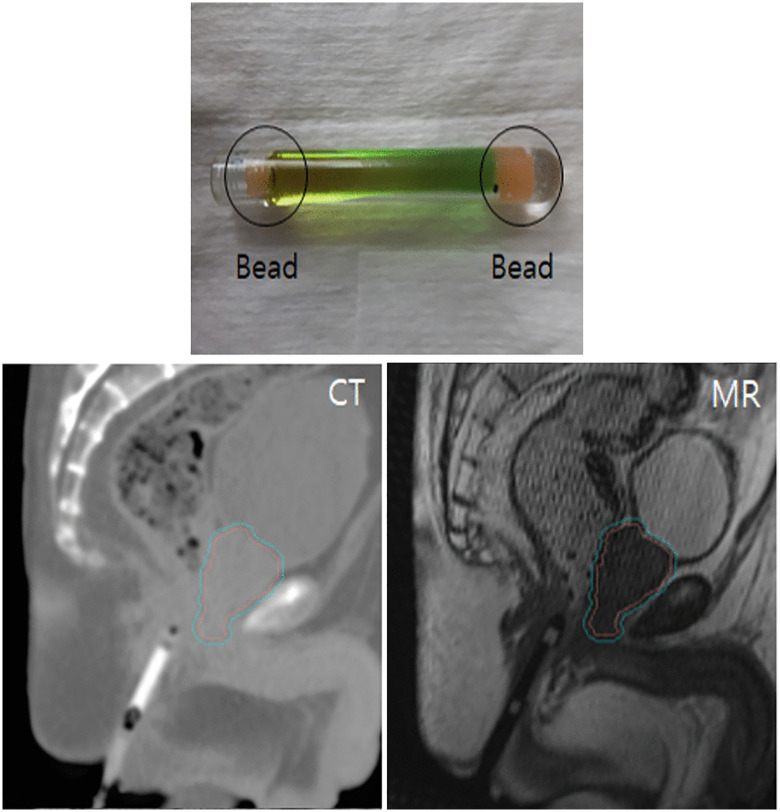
Final product of rectal in vivo dosimeter with MRI marker (upper row) and CT and MR image when the rectal dosimeter was applied to the patient (lower row).
